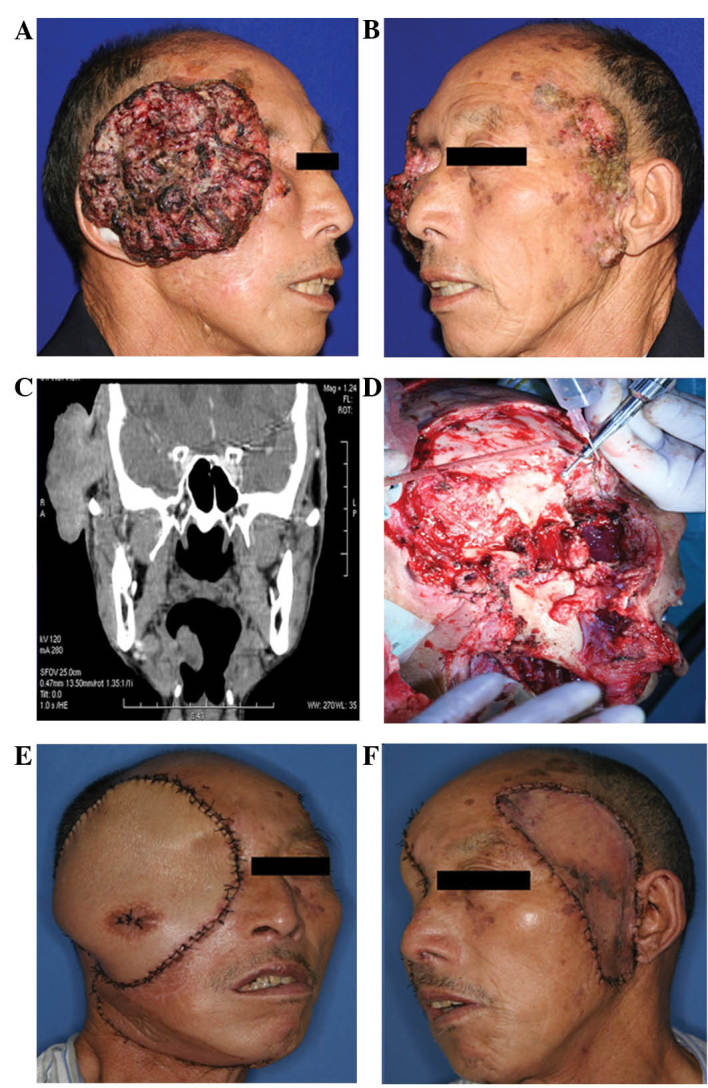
Clinical signs and treatment of a giant tricholemmal carcinoma with recurrence/multiple lesions. (A) Pre-operative image showing a giant lesion on the right temple. (B) Pre-operative image showing lesions on the left temple. (C) Pre-operative computed tomography image in the coronal plane. (D) Intraoperative image showing the appearance following radical resection of the lesions. (E) Post-operative image of the right-sided lesion taken 1 week later. (F) Post-operative image of the left-sided lesions taken 1 week later.
